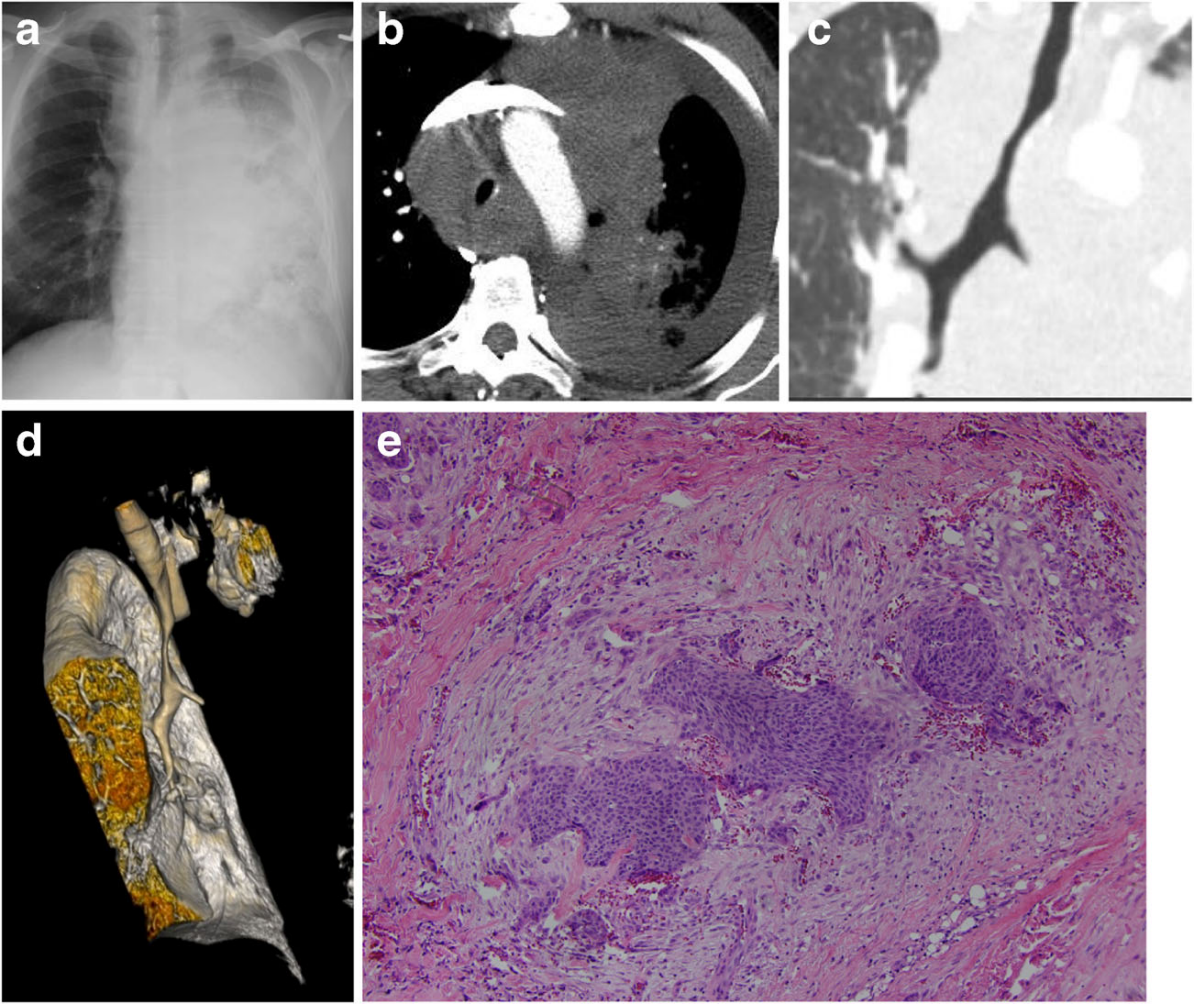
Sixty-one-year-old man with stage IV small cell lung cancer and diffuse involvement of the trachea. a Frontal radiograph, demonstrating extensive involvement of the left hemithorax. b Axial, c coronal, and d 3D VR CT images reveal direct invasion and extrinsic compression of the trachea with rightward deviation by the lesion and lymph node mass. e Pathology reveals invasive squamous cell carcinoma with desmoplastic stroma
